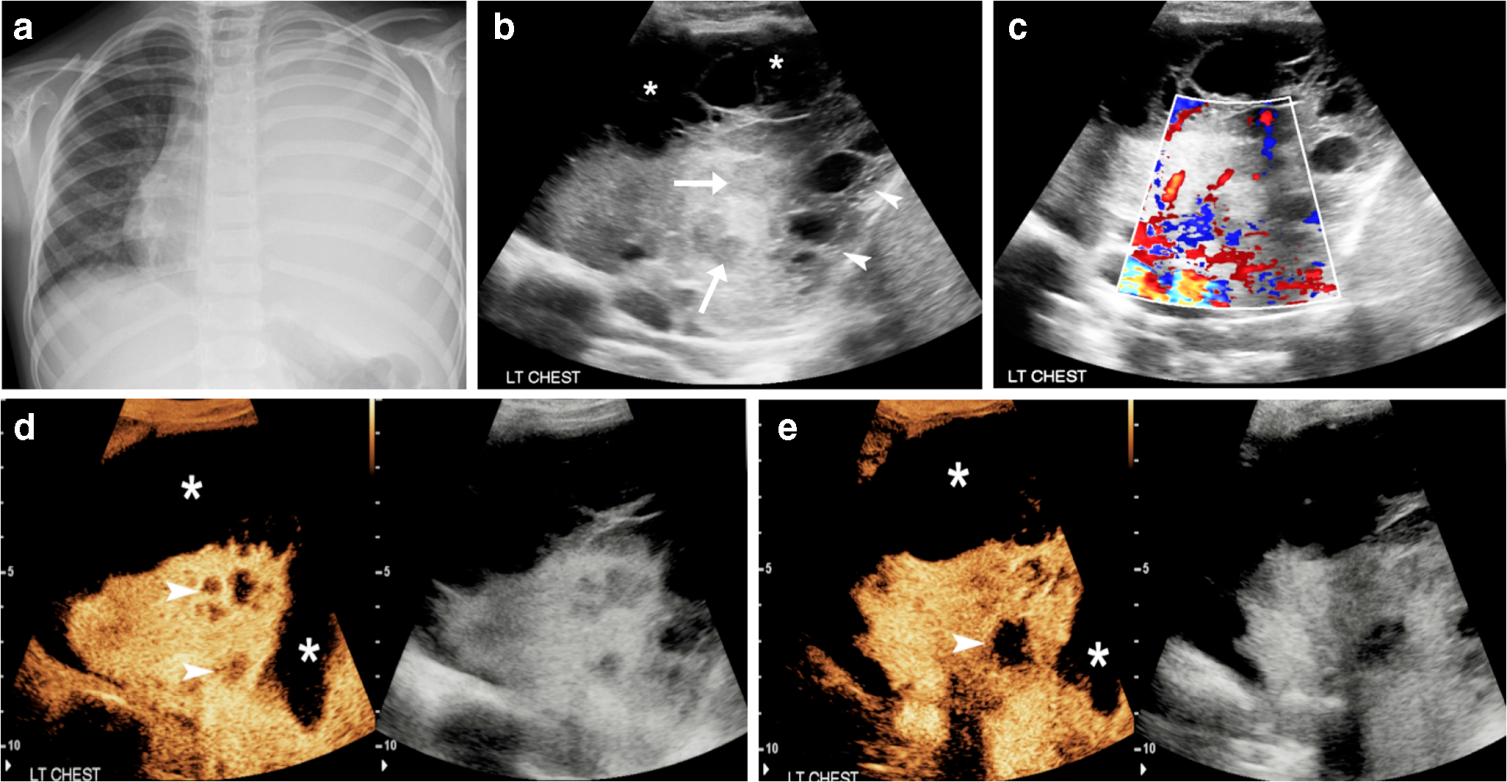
Complicated pneumonia in a 5-year-old girl. a Posteroranterior chest radiograph shows complete opacification of the left hemithorax, with no visualization of the left hemidiaphragm and the left cardiac border. The cardiac silhouette has been displaced to the right. b Gray-scale US in coronal plane through the left lung shows consolidated lung parenchyma with heterogeneous appearance containing some ill-defined hypoechoic areas (*arrows*). Adjacent to the consolidated lung there is complex multiloculated pleural effusion (*asterisks*), in keeping with empyema, which extends in a sub-pulmonary location (*arrowheads*). It is difficult to delineate the exact extent of the empyema and evaluate the adjacent consolidated lung because of the complex echogenicity of the empyema. c Color Doppler US in coronal plane through the left lung. Image quality is degraded by overwriting artifacts from movement and breathing. There is some blood flow signal within the consolidated lung; however, exclusion of necrotizing pneumonia is not possible. d, e Contrast-enhanced ultrasound (CEUS) dual-screen mode with simultaneous display of contrast (*left*) and gray-scale (*right*) modes from superior (d) to inferior (e) aspects of the left hemithorax in coronal plane. There is heterogeneous enhancement of the consolidated lung, which is clearly distinguished from the adjacent non-enhancing parapneumonic effusion. Within the consolidated lung there are multifocal non-enhancing areas (*arrowheads*) suggestive of avascular lung tissue that is not surrounded by thickened or rim-enhancing wall, in keeping with necrotizing pneumonia rather than abscesses. CEUS also significantly improved the delineation of the overall volume of the parapneumonic effusion (*asterisks*)
